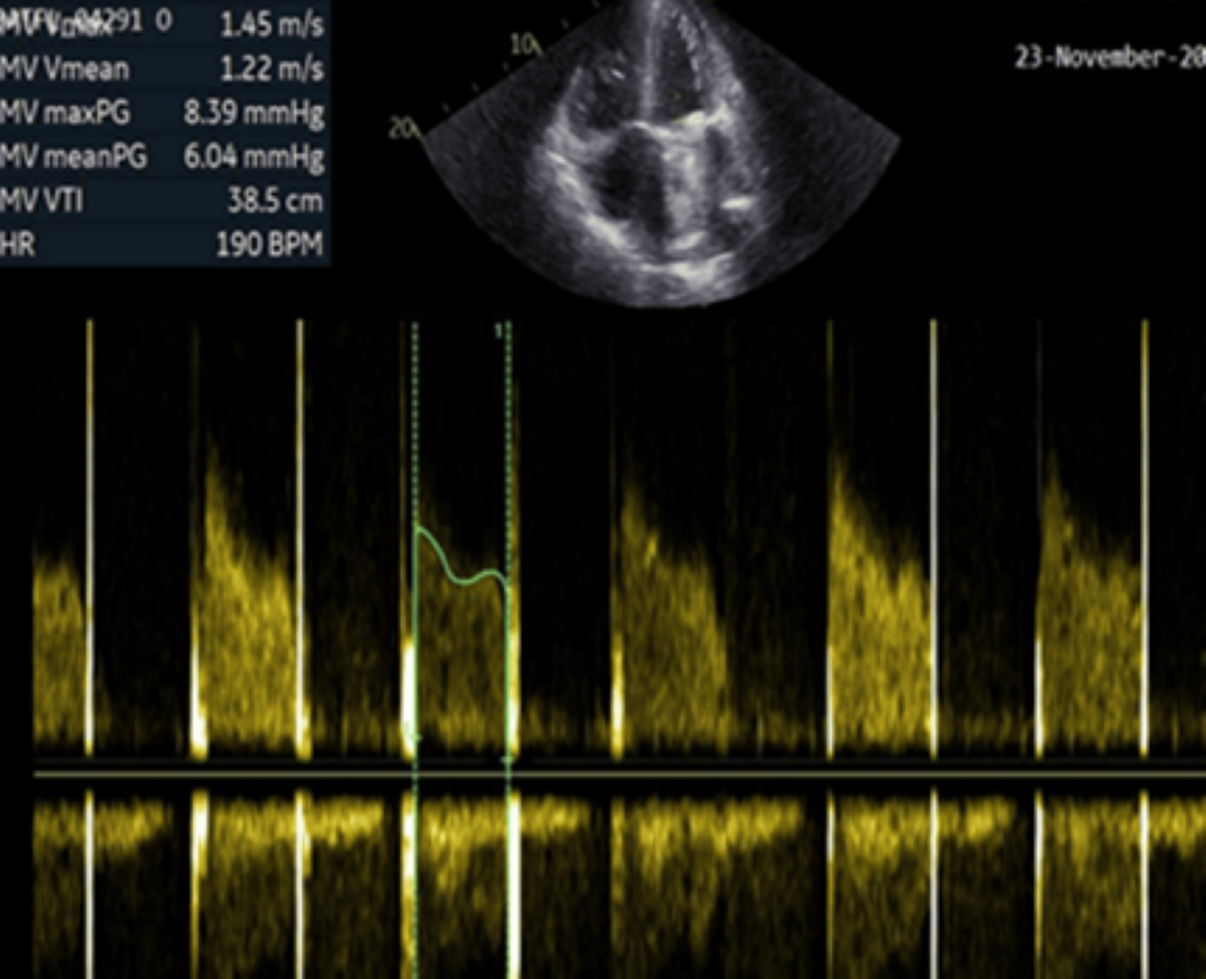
Spectral Doppler across the mitral prosthesis post lytic therapy
